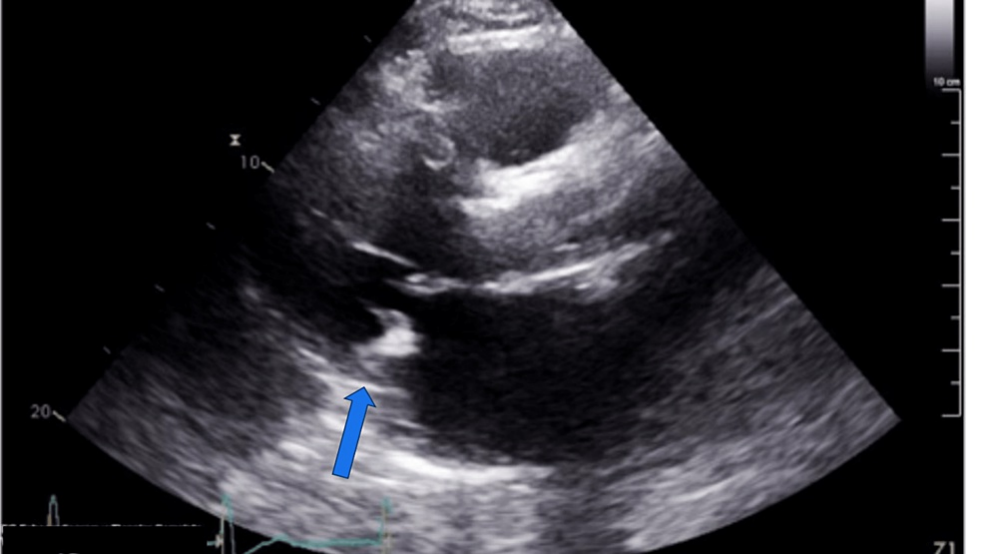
Parasternal long-axis view on TTE showing TAVR (blue arrow), no vegetation, and no para-valvular leak (not shown in the still image). TTE: trans-thoracic echocardiogram; TAVR: transcatheter aortic valve replacement.
